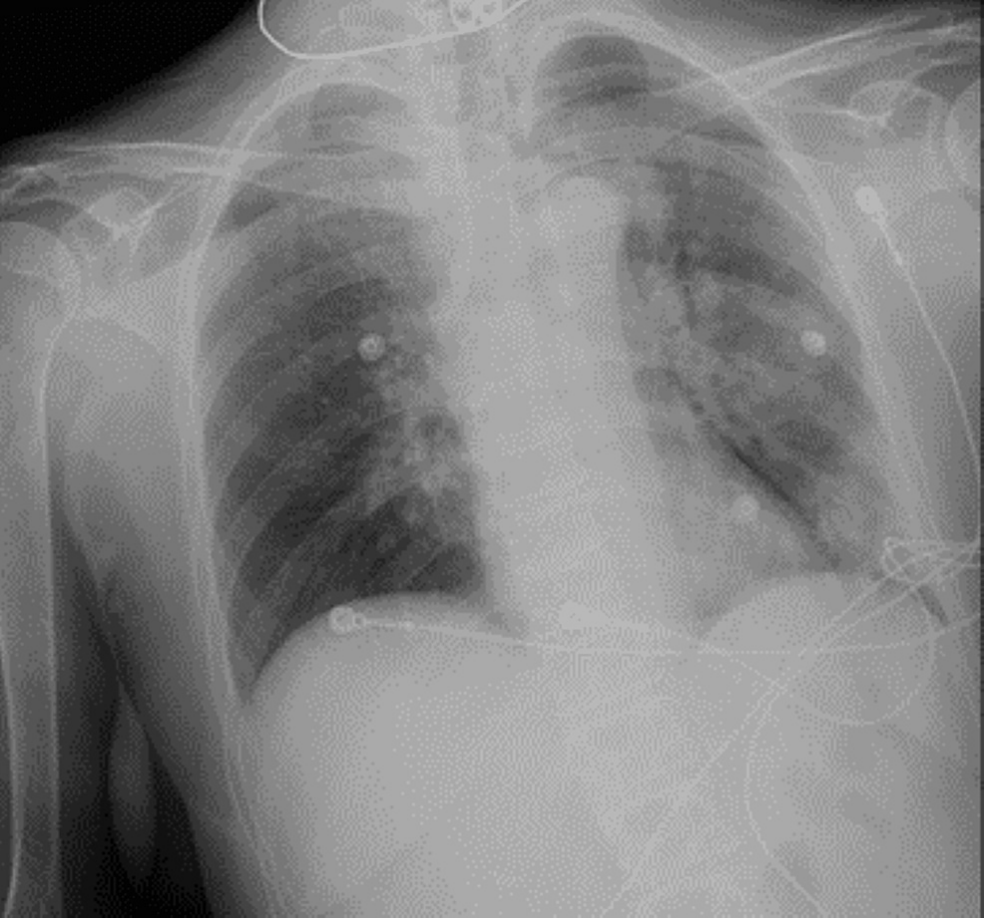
CXR showing left lower lobe pneumonia. CXR: Chest X-ray
